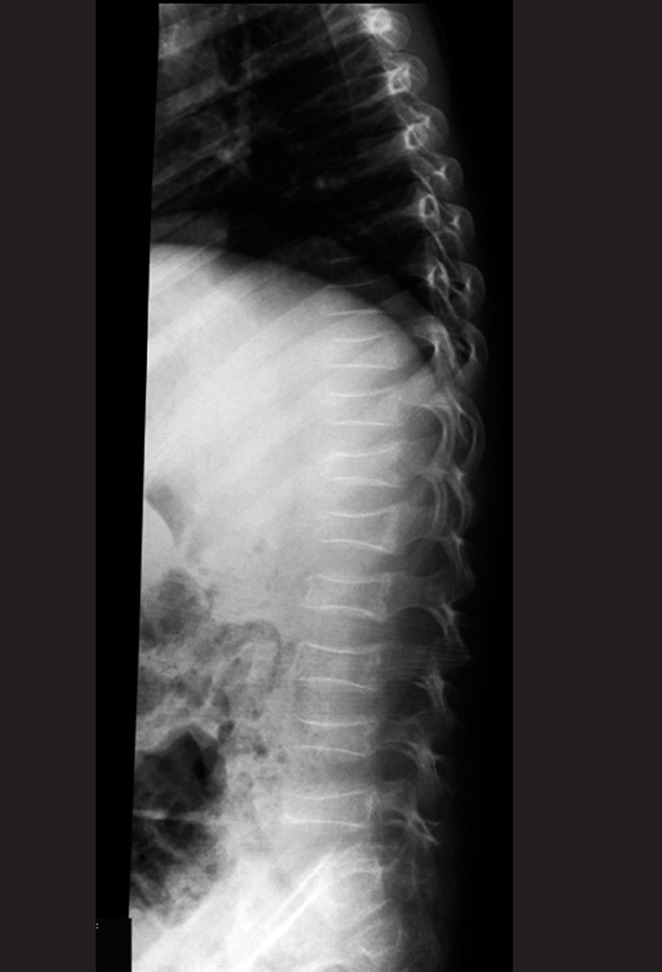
A 4-year-old girl with ALL presenting with back pain and pancytopenia Lateral thoracolumbar X-ray reveals multiple collapsed vertebrae.
